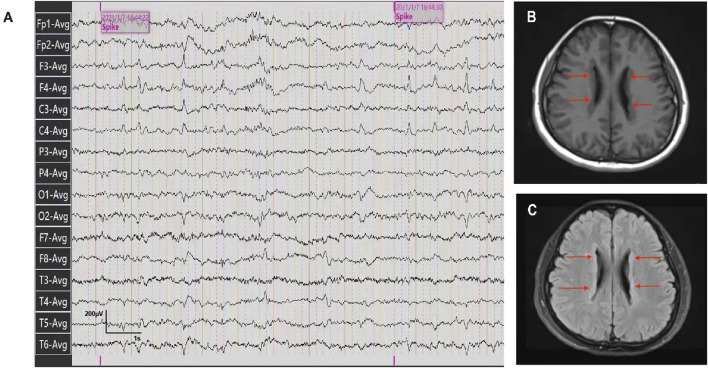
Ambulatory electroencephalogram and cranial MRI images of the patient. (A) Ambulatory electroencephalogram detected epileptiform discharges. (B, C) T1-weighted and T2 FLAIR images of the patient detected the bilateral periventricular nodular.
